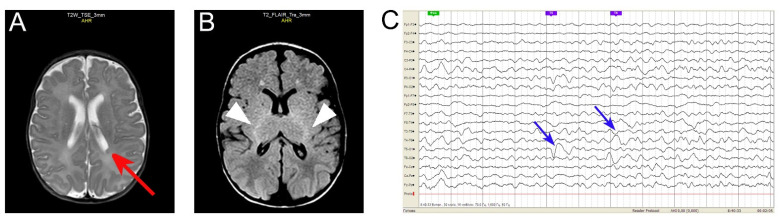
Brain MRI and EEG of the *GNAO1* Gln52Arg patient. (A,B) Brain MRI ((A): axial T2 weighted sequence, (B): axial FLAIR) reveal left posterior periventricular nodular heterotopia (PVNH, arrow in (A)) and hyperintensity of the posterior limb internal capsulae (arrowheads in (B)). (C) EEG reveals left posterior temporoparietal slowdowns (arrows).
